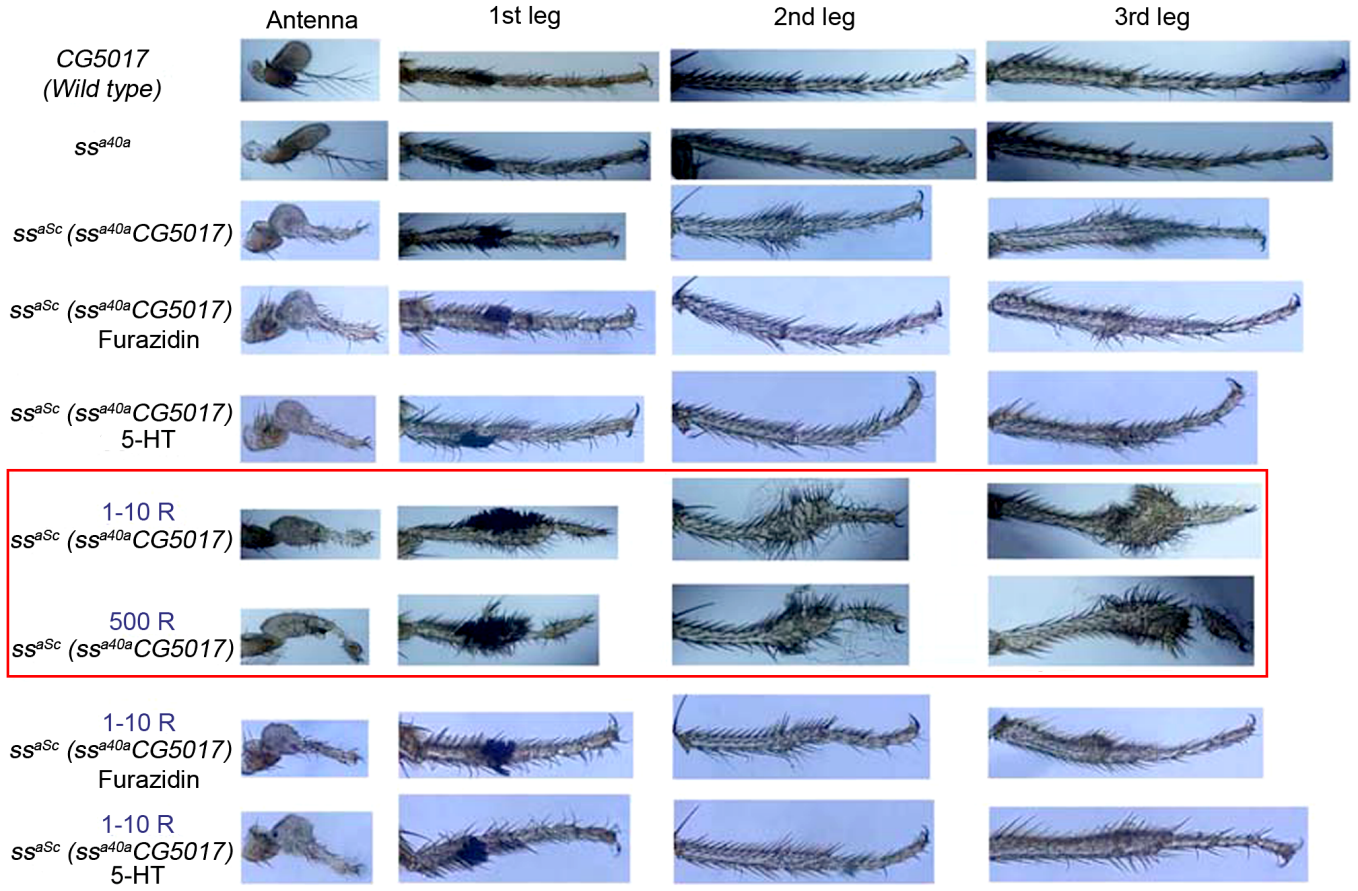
Wild type and mutant antenna and leg in flies in normal conditions and following irradiation. Simultaneous combination of mutations at both *ss* and *CG5017* loci increases sensitivity to even low doses of X-ray radiation (1 and 10 R), which is manifested as an increase in the mutant phenotype (photographs within the red frame).
